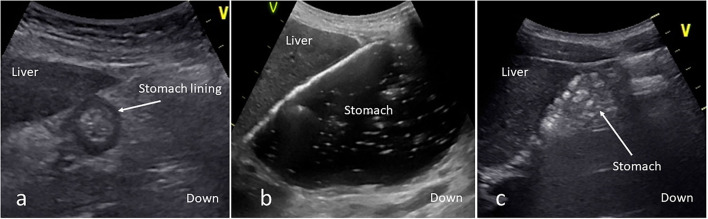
A gastric point-of-care ultrasound [(a) empty stomach; (b) full stomach with liquid; (c) full stomach with solid]. On a sagittal/parasagittal ultrasound scanning plane of the epigastric area, the stomach is visualized under the liver. The “v” mark indicates the probe cursor.
